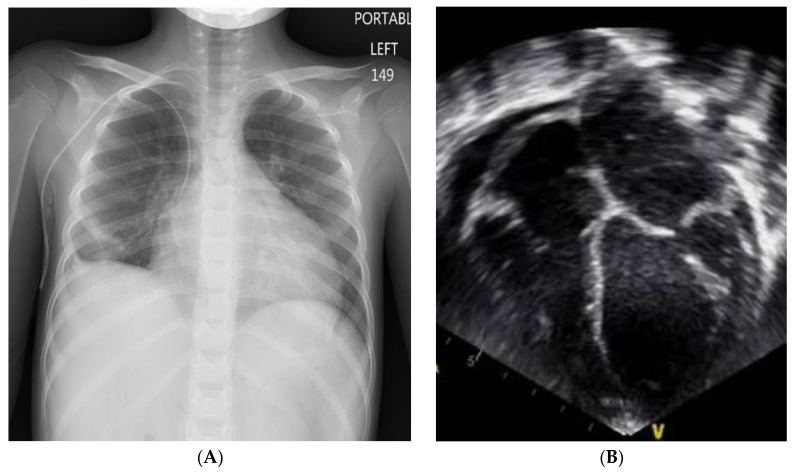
Myocardial recovery after VAD implant. (A) Chest x-ray image prior to VAD implant showing cardiomegaly. (B) Prior to VAD implant, trans-thoracic echocardiogram in the apical four-chamber view showing a moderately dilated left atrium (LA) and left ventricle (LV) with ventricular wall thinning and moderately depressed systolic function with a shortening fraction of 16% and calculated LVEF of 38%.
